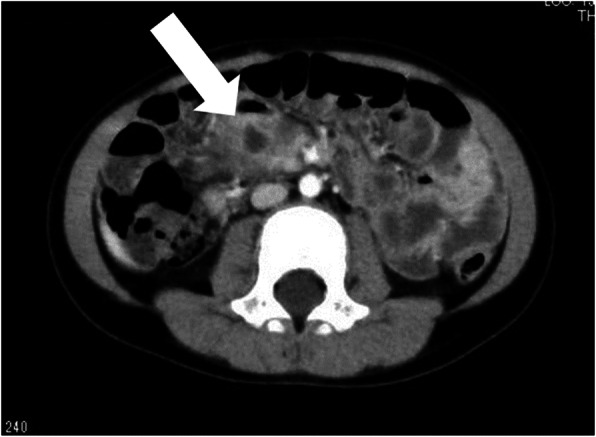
Follow-up computed tomography (CT), case 2. CT shows the remaining duplication cyst around the end of the ileum (white arrow)
